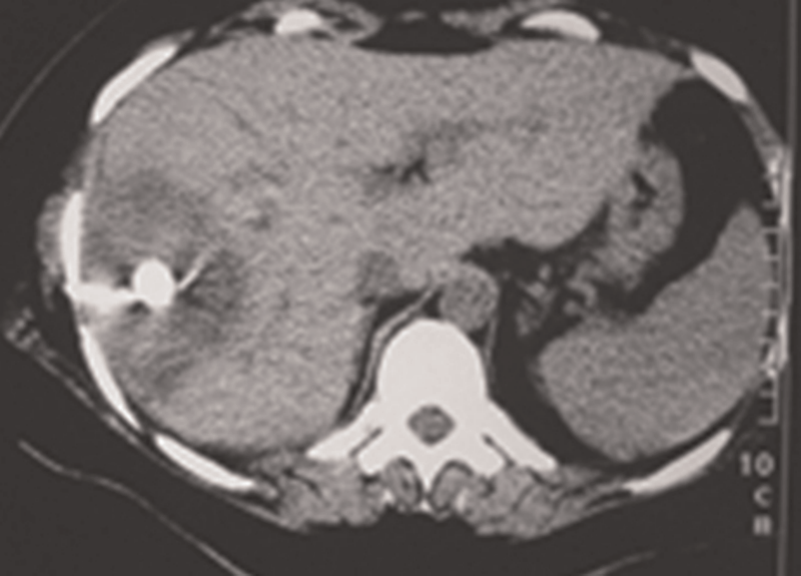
CT scan during percutaneous drainage under CT guidance reveals that the drainage catheter is in good position within the abscess cavity.
